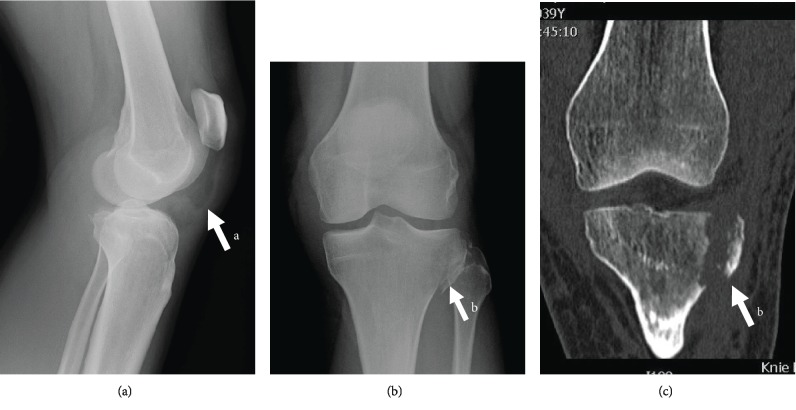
Preoperative X-rays: anterior-posterior (a), lateral (b), and coronal (c) view CT. Note the patella alta with a Caton Deschamps index of 1.5 (A) as an indirect sign of the PT rupture, as well as tibial plateau fracture (B).
